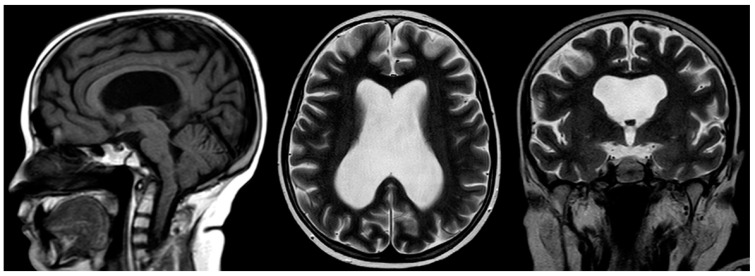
Sagittal T1-weighted, and axial and coronal T2-weighted MRIs of a 12-year-old male with confirmed Fanconi anemia. Mild basilary impression is noted with angulation at the cranio-cervical junction, small-sized pituitary gland, moderate supratentorial ventriculomegaly, and an absent septum pellucidum with box-like configuration of the lateral ventricles compatible with septo-optic dysplasia.
